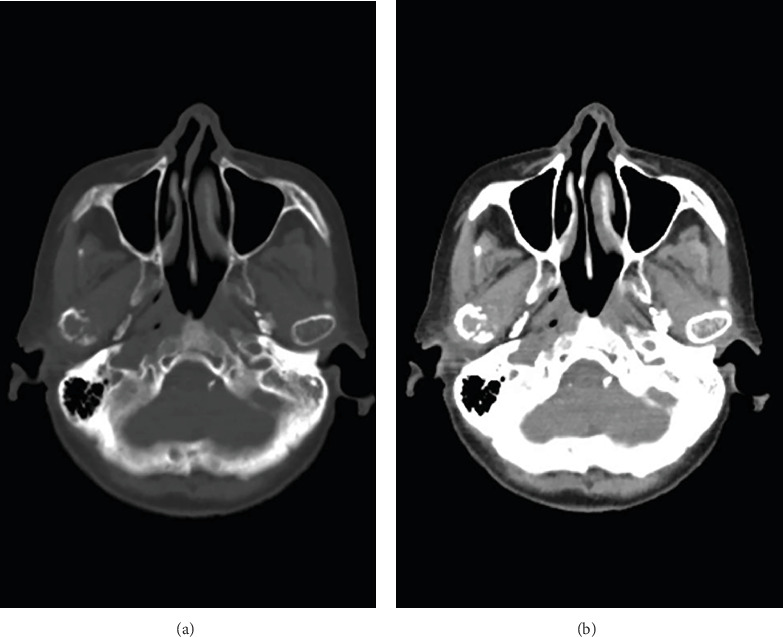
Axial computed tomography at first examination. (a) Hard tissue imaging. (b) Soft tissue imaging. An osteolytic lesion is observed in the right mandibular condyle.
